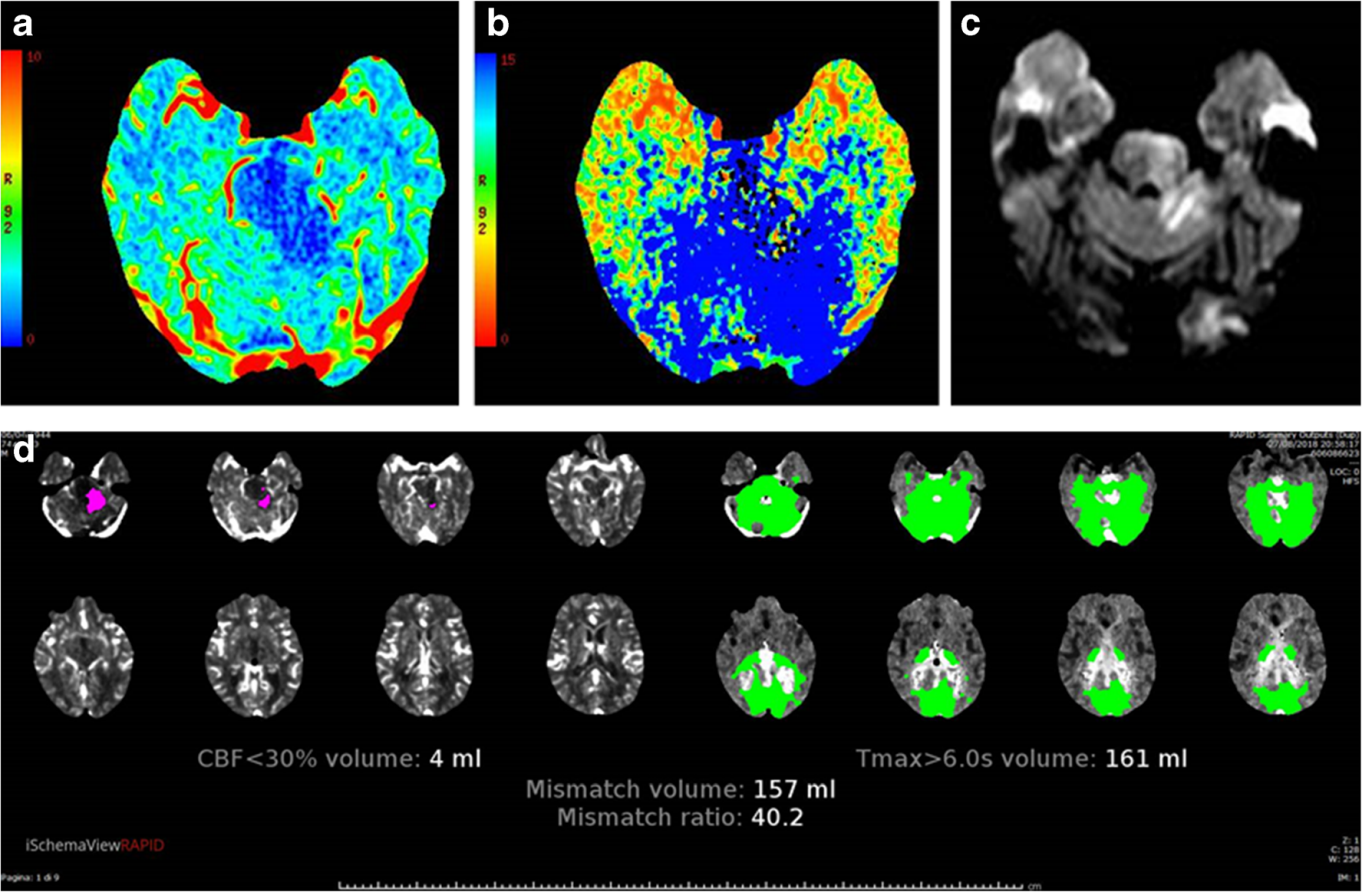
Left cerebellar and mesencephalic ischaemic changes recognised as core and penumbra, respectively, on CBV (a) and MTT (b) colour-coded CT perfusion 4D maps; the presence of ischaemic core was confirmed by DWI-MRI performed immediately after to better evaluate the involvement of mesencephalon; the automatic RAPID mismatch map (d) accurately depicted the extent of ischaemic core and penumbra
